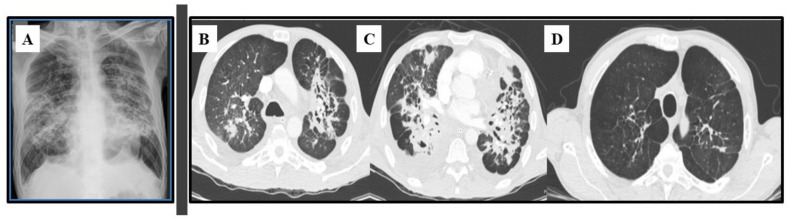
Lung images of a 53-year-old male patient with chronic paracoccidioidomycosis. (A) Plain chest radiograph with bilateral, parahilar, and symmetrical interstitial alveolar infiltrate (“butterfly wing” pattern); (B,C) Axial thoracic CT at diagnosis with multiple bronchiectasis associated with areas of irregular parenchymal opacities, and diffuse nodules (some of them excavated); (D) Axial thoracic CT at the end of antifungal therapy: areas of emphysema with traction bronchiectasis, retractable striae, and central architectural distortion, predominantly peribronchovascular, indicating fibrosis.
